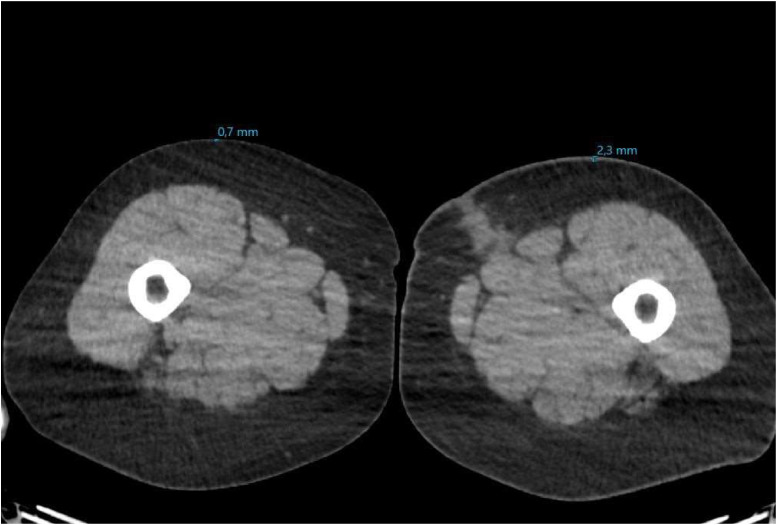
Example of skin thickness measurements using PET-CT
